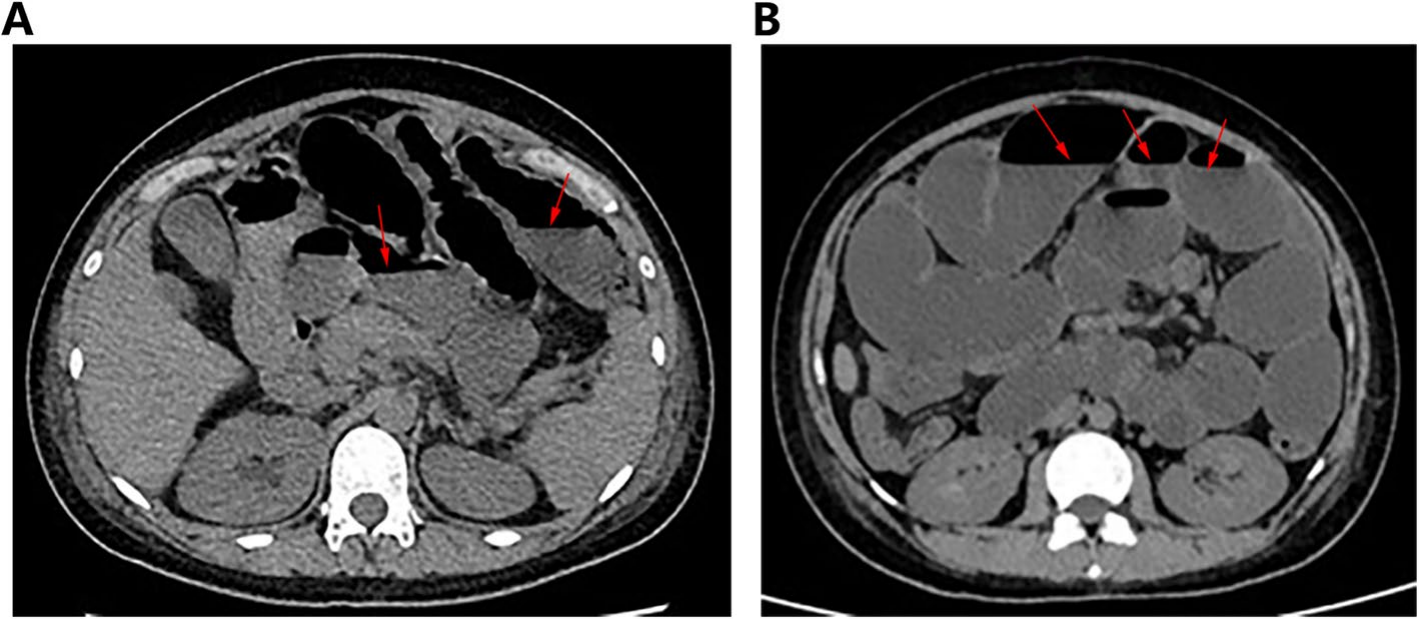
CT images show typical manifestations of intestinal obstruction. A the CT image for the first time revealed dilated small bowel with the widest diameter of 45 mm suggestive of small bowel obstruction; B the repeated CT image revealed stable dilated small bowels with the widest diameter of 53 mm, with no signs of mechanical obstruction, bowel necrosis, or perforation. Red arrows point to the typical air-fluid level of the bowel
